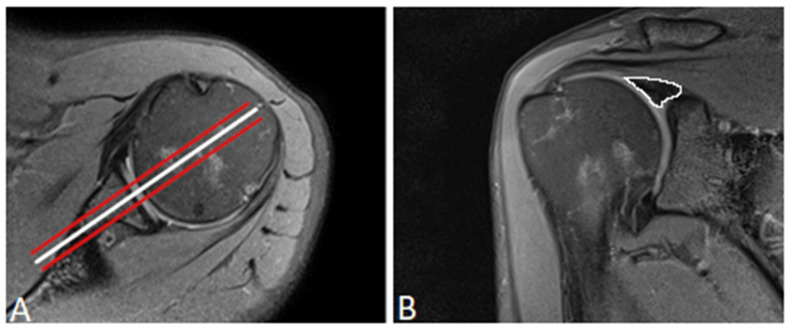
(A) Axial proton density-weighted, fat-saturated magnetic resonance image demonstrating the three oblique coronally oriented sections used for ROI placement: one midsection through the glenoid fossa (white) as well as one adjacent slice, anteriorly and posteriorly (red). (B) Coronal proton density-weighted, fat-saturated magnetic resonance image depicting the manually drawn ROI (white) outlining of the entirety of the glenoid labrum on a midcoronal section. The ROI was copied into the color-coded T2 mapping sequence.
